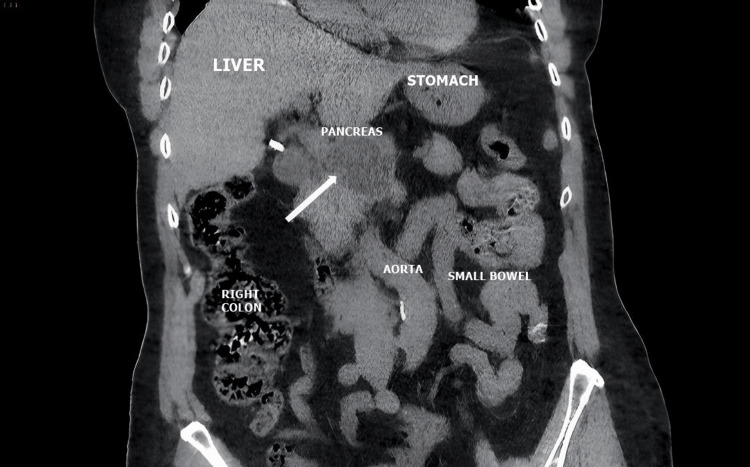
Pancreatic hydatid cyst (indicated by the white arrow): CT images in the coronal section (personal case study).
